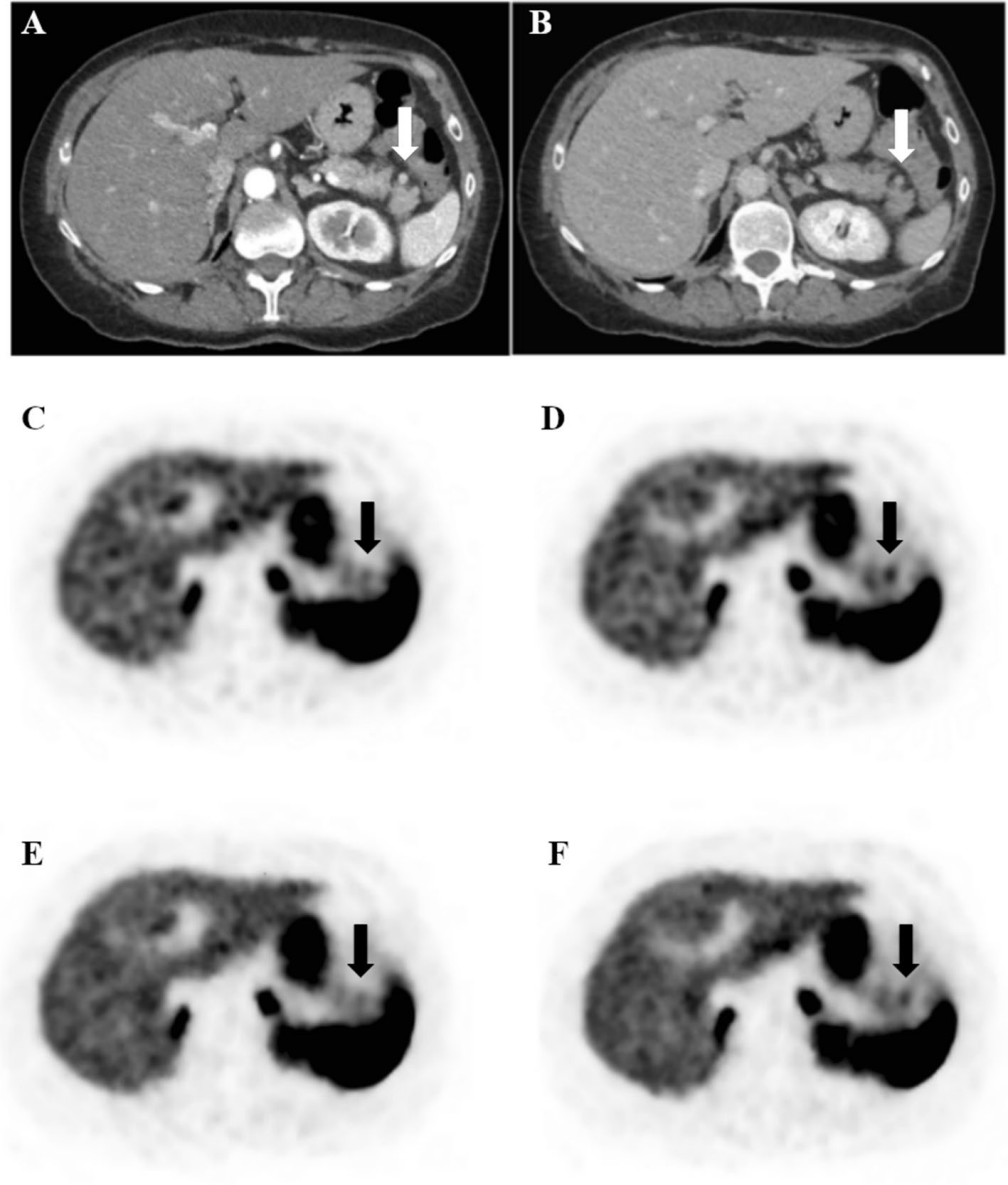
^68^Ga-DOTATOC PET/CT showing an intrapancreatic accessory spleen mimicking a pancreatic neuroendocrine tumor. Contrast-enhanced CT image in portal-venous phase showing the lesion in the pancreatic tail (a). Contrast-enhanced CT image in arterial phase showing the enhancing lesion in the pancreatic tail. The lesion is characterized by mild ^68^Ga-DOTATATE uptake, more evident in OSEM-DDG (d) and BSREM-DDG (f) images (SUVmax 8.6 and 7.6, respectively) compared with OSEM (c) and BSREM (e) images without DDG (SUVmax 6.4 and 6.2, respectively). The PET volume of the accessory spleen is smaller in OSEM-DDG and BSREM-DDG images (980 mm^3^ and 814 mm^3^, respectively) compared with OSEM and BSREM images without DDG (1400 mm^3^ and 1520 mm^3^, respectively).
